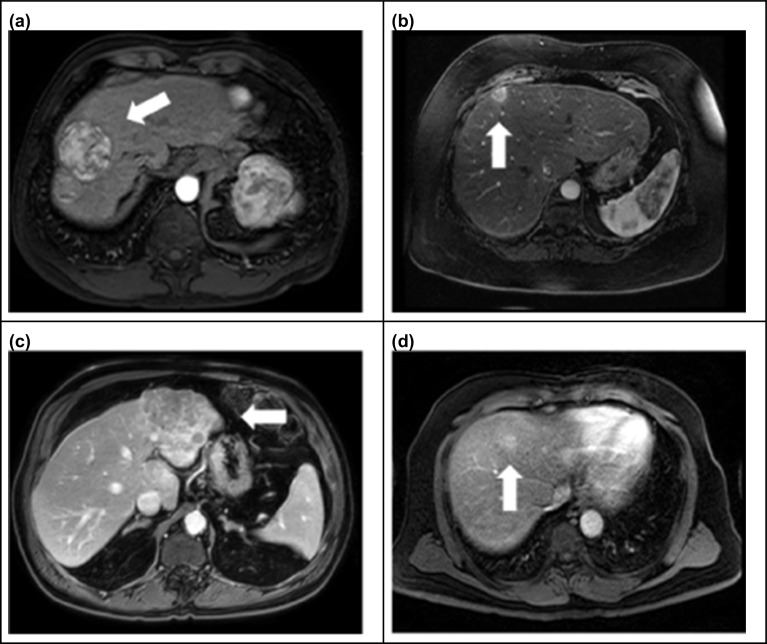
Example HCC lesions as seen on dynamic post-contrast T1-weighted fat-saturated MRI images. a Large, well defined (code 46.1, corresponding to patient #46, lesion #1). b Small, well defined (39.3). c Large, poorly defined (49.1). d Small, poorly defined (55.4). Each image is displayed in the optimum visualization window and phase used for segmentation
